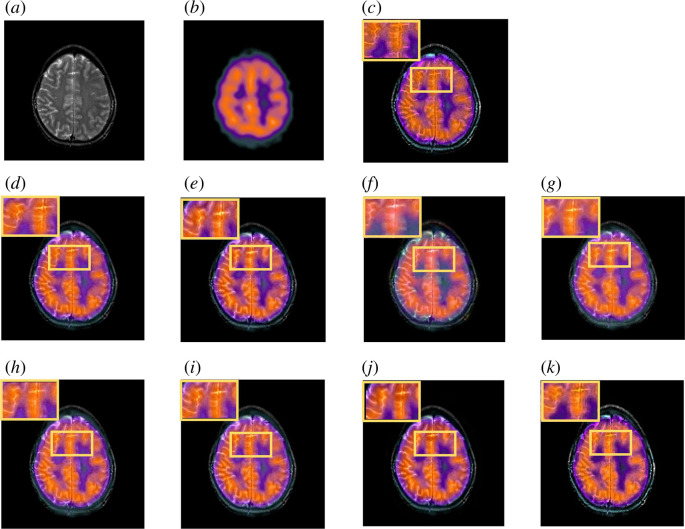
Comparison of fused image on the MRI-SPECT from metastatic bronchogenic carcinoma image dataset. (*a*) Original MRI image. (*b*) Original SPECT image. (*c*) MLMG-PCNN [16]. (*d*) JBF [18]. (*e*) Local extreme map GF [39]. (*f*) LRD [7]. (*g*) ACOF [40]. (*h*) MDHU [41]. (*i*) FGF [42]. (*j*) Fusion based on phase congruency [43]. (*k*) Proposed.
